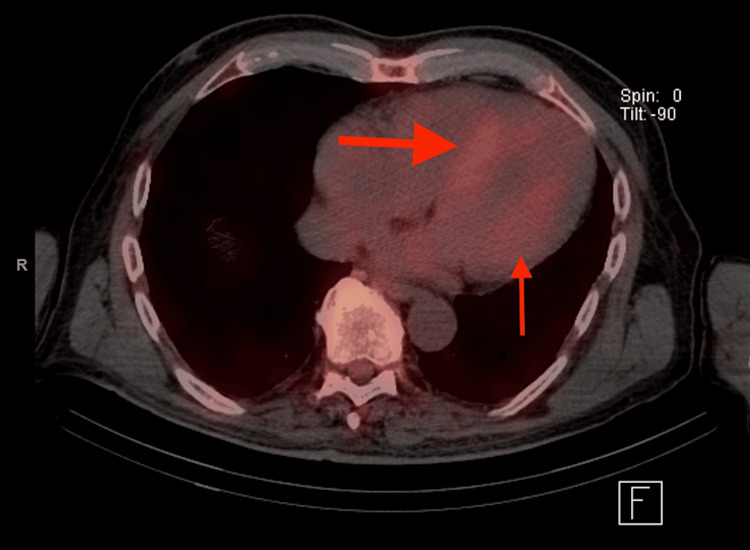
The patient’s technetium-99m pyrophosphate nuclear scintigraphy shows uptake limited to the myocardium (red arrows) on single-photon emission computerized tomography.
